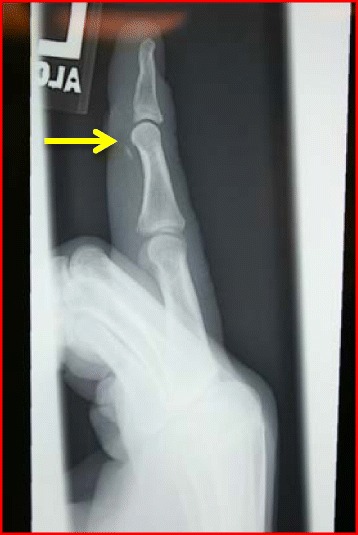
Lateral radiograph of a ring finger of a patient whose finger got caught on a basketball net showing an FDP bony avulsion fracture with the fragment caught up distal to the A4 pulley level (*yellow arrow*)
